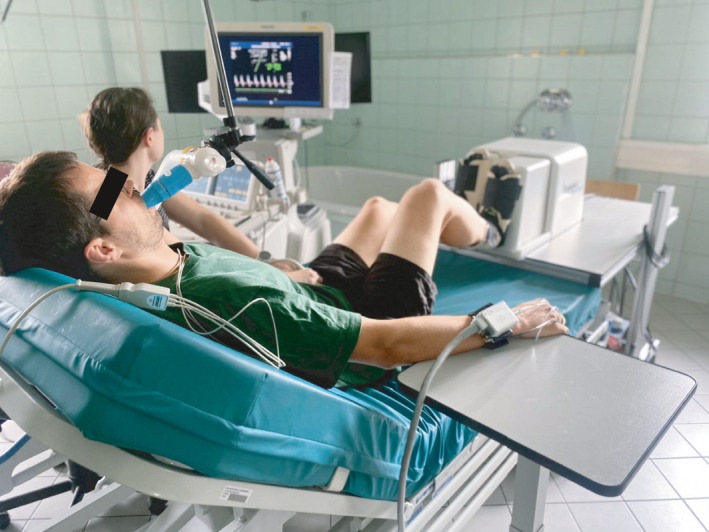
Experimental set up of a participant performing stepping exercise during measurement of femoral artery blood flow with Doppler ultrasound
